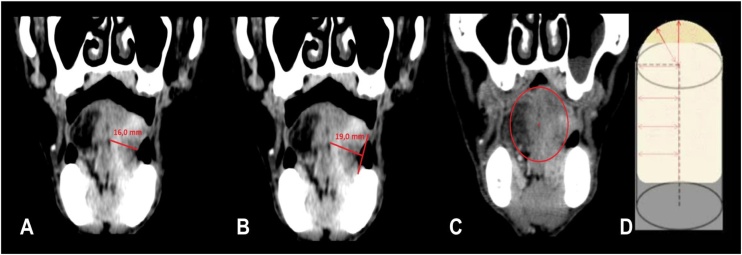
Demonstration of the method used to measure tumor thickness and depth of invasion by computed tomography (coronal images) proposed by the AJCC Cancer Staging System. (A) Demonstration of the method used to measure tumor thickness: measurement used in the old version of TNM classification for oral cavity cancers (7th edition); (B) Demonstration of the method used to measure the depth of invasion (extrapolation of the standardized method in anatomopathological samples by AJCC described at the new TNM ‒ 8th edition). The measurement is based on the conic format of the tongue; (C) An imaginary line is drawn between the two edges of the intact mucosa; (D) Another line is drawn from the point of greatest invasion to the first line, taking as reference the central point of the tongue, the latter, then, will be the measure of depth of invasion).
